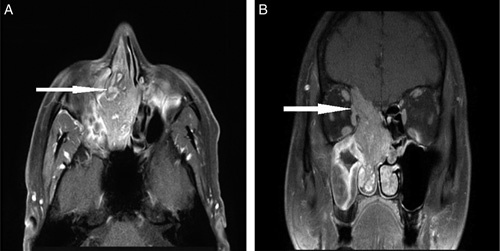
Axial section image of MRI nose and PNS on T1W sequence post enhancement (A), Coronal section image of MRI nose and PNS on T1W sequence post enhancement (B). PNS, paranasal sinuses.
